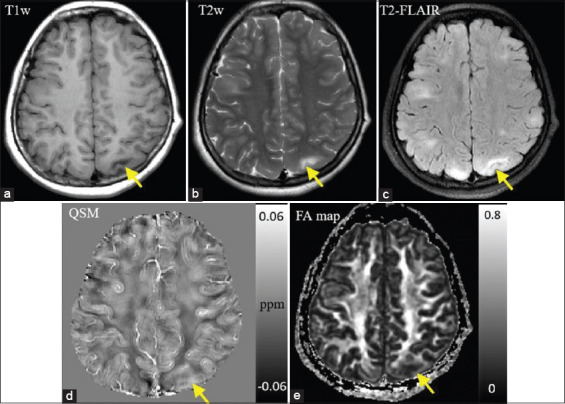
(a-e) Example of cortical/subcortical nodules from a 16-year-old female patient. The tuberous sclerosis complex lesions appeared hypo- or iso-intense on T1-weighted image, hyperintense on T2-weighted image, and T2-fluid-attenuated inversion recovery. The lesions had hyperintense on quantitative susceptibility mapping and hypointense on fractional anisotropy map.
